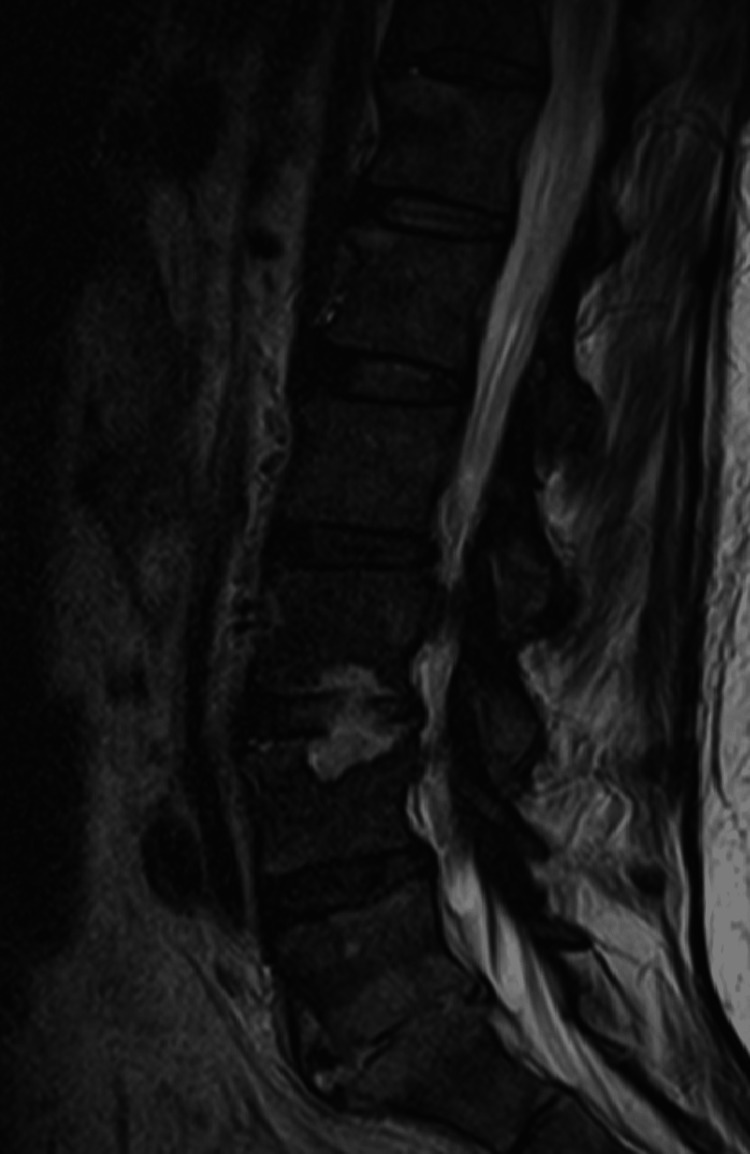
MRI right before the beginning of the treatment (sagittal view)
